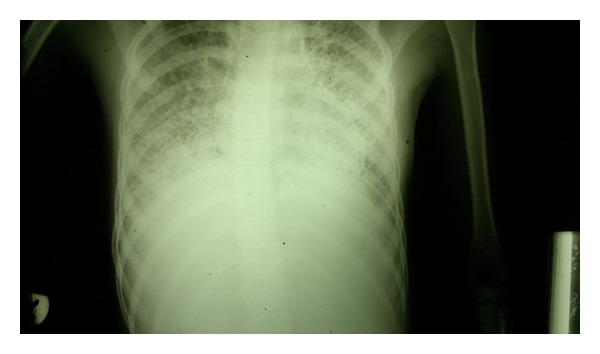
A chest radiograph showing interstitial lung disease.
